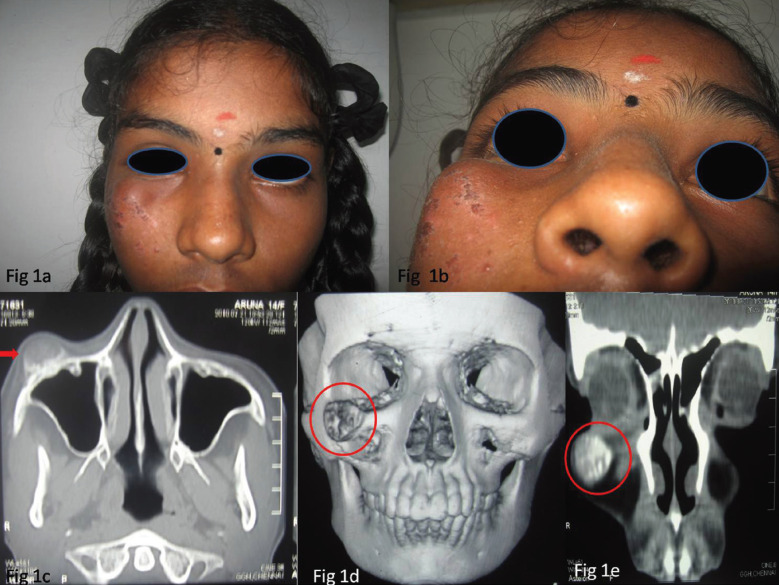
a- Profile view of the patient showing Extraoral swelling. b- Extra oral view showing ulcerated skin. c- Computed Tomography(CT) Axial View showing lesion of mixed densities in the infraorbital rim. d-CT -3D reconstruction image showing lesion of mixed densities in the infraorbital rim. e-CT-coronal view showing lesion of mixed densities in the infraorbital rim.
